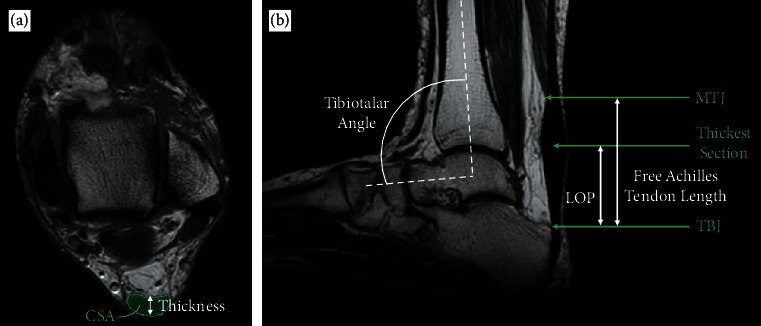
T1-weighted MRI scan demonstrating the measurements made. (a) The axial image with the cross-sectional area (green) and thickness (white arrow) of the tendon. (b) The sagittal image showing the three locations that the cross-sectional area (CSA) and thickness measurements were made (green arrows) as well as the location of pathology (LOP), free Achilles tendon length and tibiotalar angle measurements (white arrows/curve).
